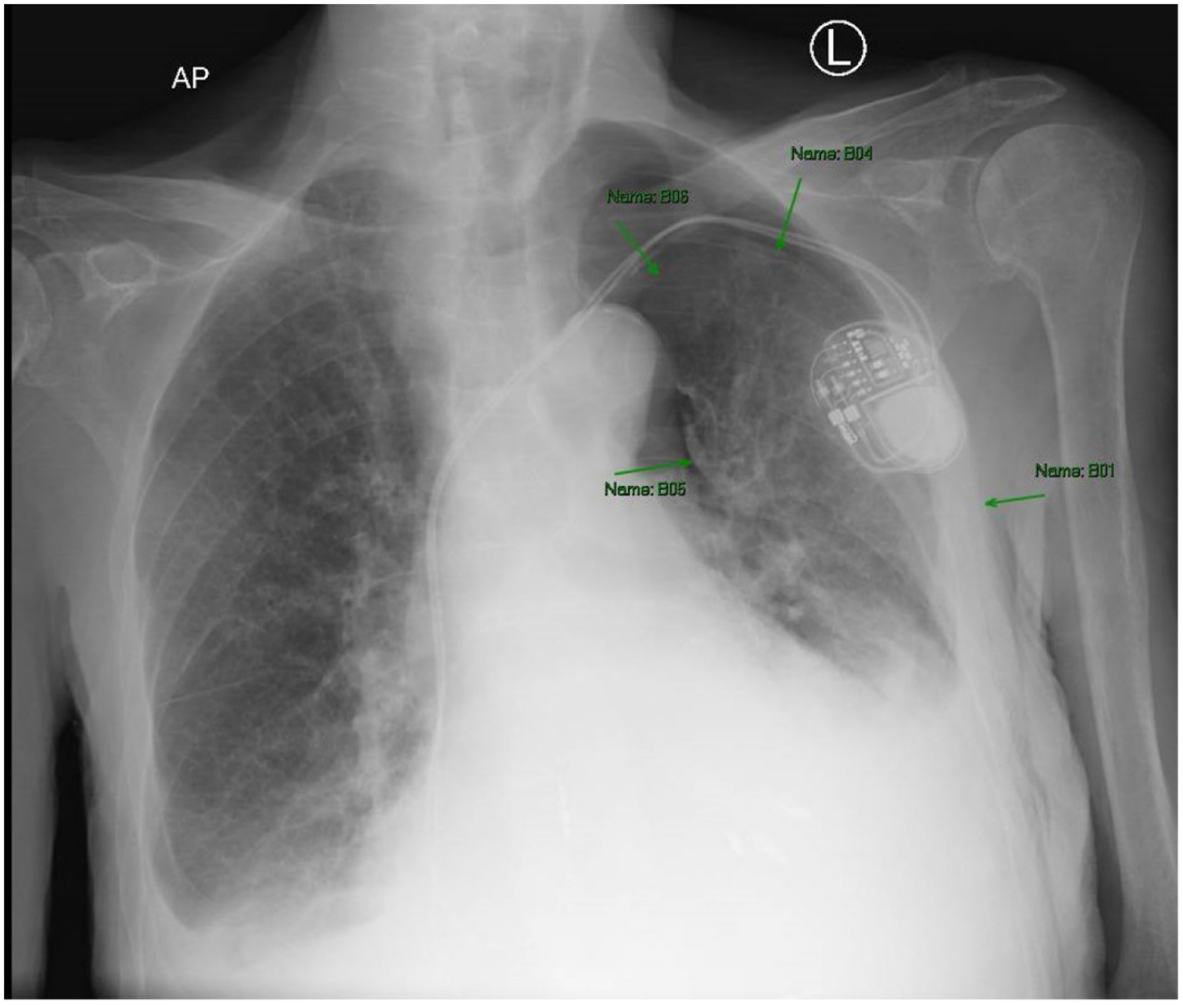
Chest radiograph with arrows pointing to left-sided moderate circumferential pneumothorax. There is a loss of symmetry, a visible lung border, and loss of lung marking superior to the lung border.
